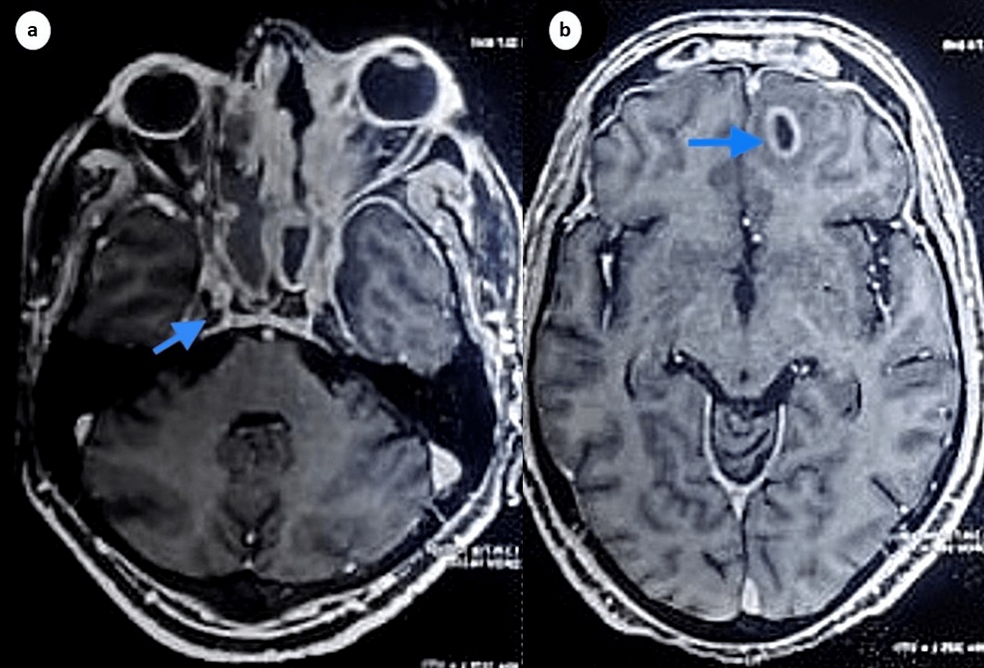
MRI brain demonstrating heterogenous enhancement of right cavernous sinus (a) and cerebral abscess in the right frontoparietal region (b).
